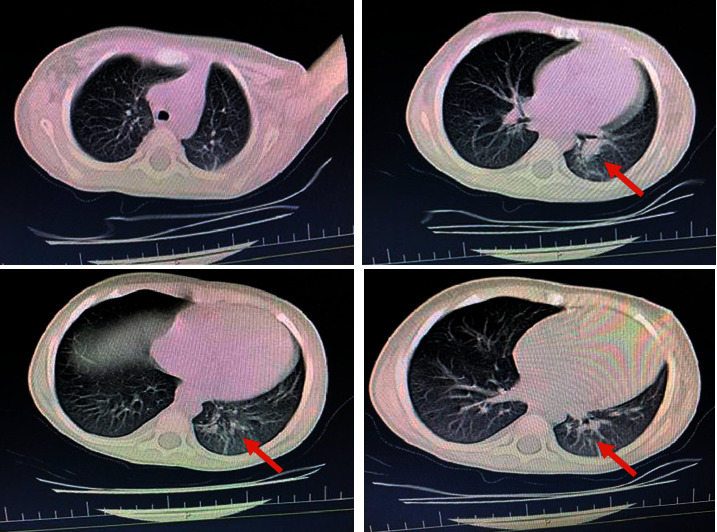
Spiral CT scan of chest without contrast.
